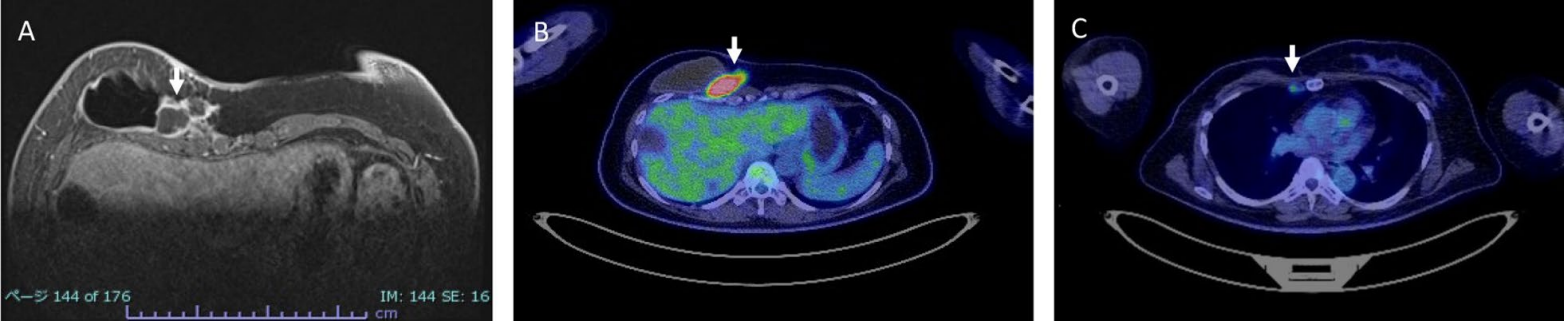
Preoperative imaging. MRI and PET–CT findings. A MRI. An irregular mass enhanced only on the margin was recognized in the inner side of the implant of right breast (white arrow). B, C FDG-PET–CT. B A mass with high FDG accumulation (SUVmax = 41.54) at the same position as on MRI (white arrow). C An internal mammary lymph node was swollen and showed mild FDG accumulation (SUVmax = 2.82), which suggested metastasis (white arrow)
